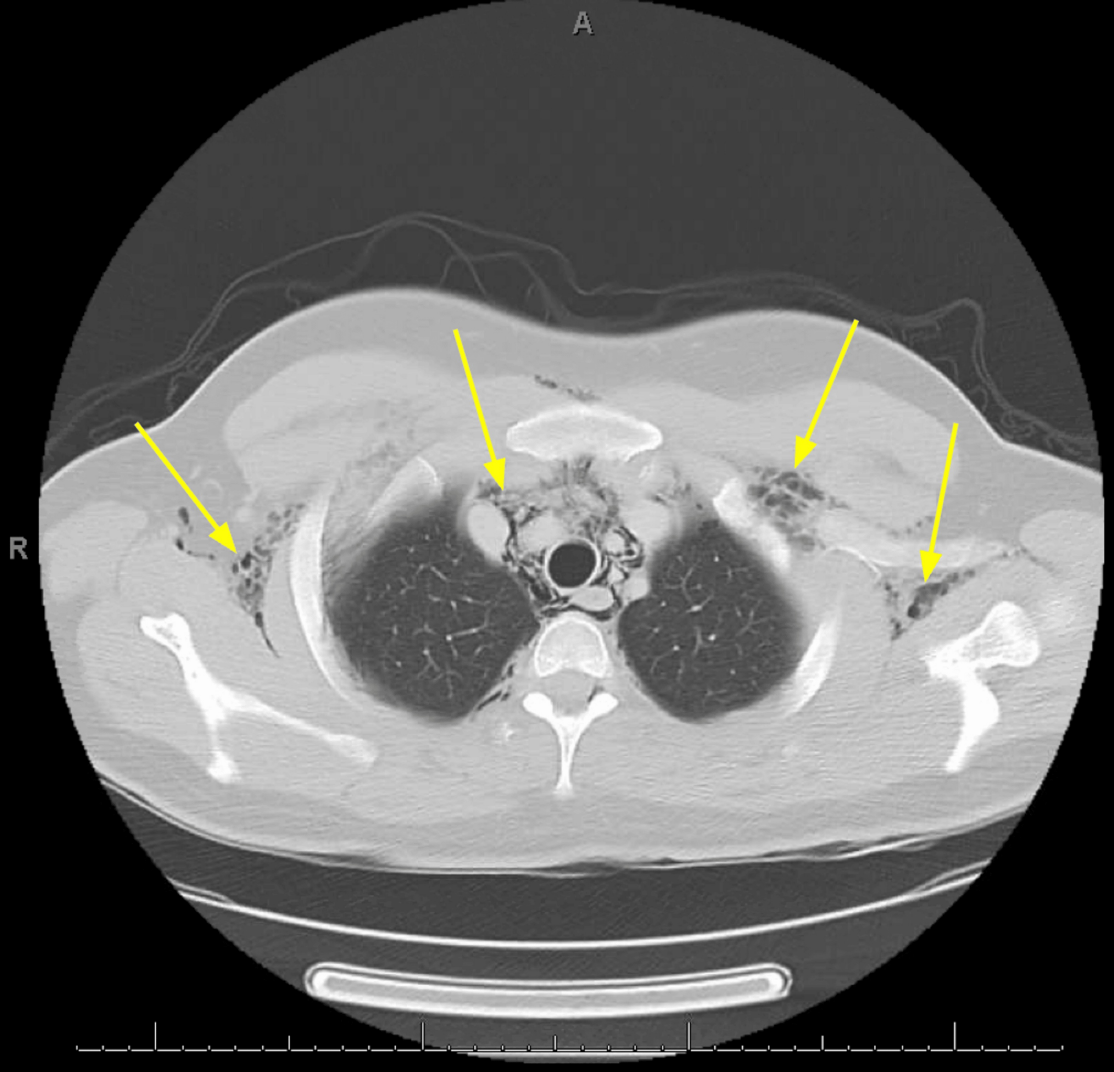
Computed tomography with contrast of the chest at the level of the superior mediastinum showing subcutaneous emphysema within the upper chest
